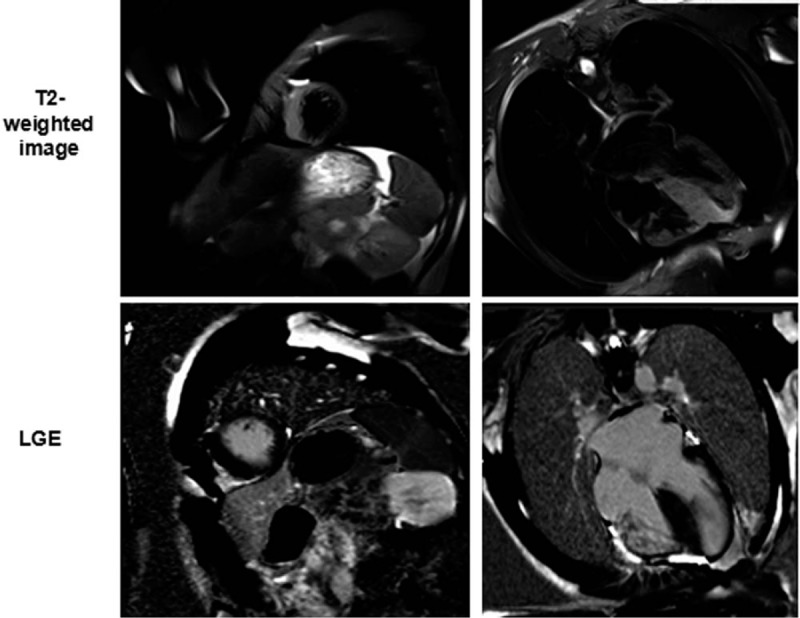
Cardiac magnetic resonance images of late gadolinium enhancement (LGE) and T2-weighted imaging in patients with hypertrophic cardiomyopathy. The LGE images (lower panel) and T2-weighted images (upper panel) are shown at the identical slice location in the same view. The areas of high T2 signal do not correspond well to those of LGE in patients with HCM.
